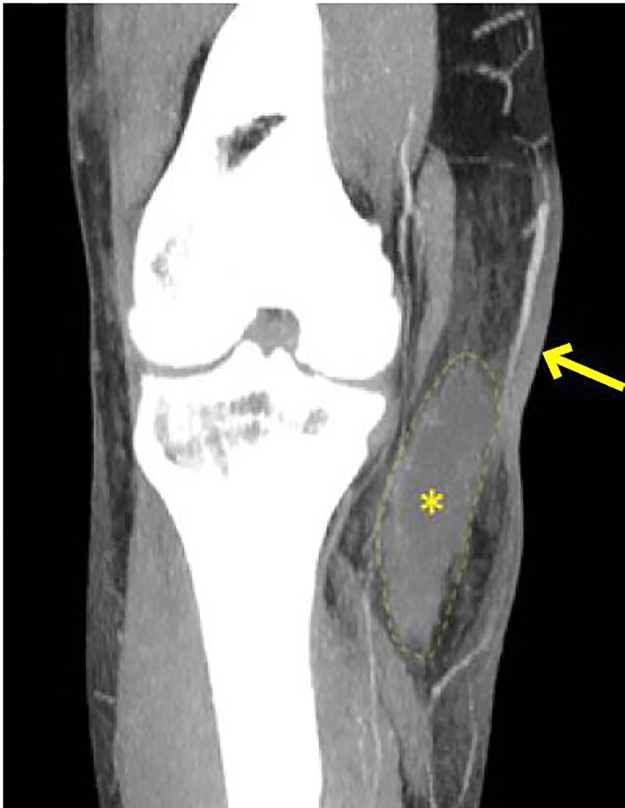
Coronal computed tomography image at level of the knee joint demonstrates an abscess (dashed outline with asterisk) in the medial subcutaneous tissues superficial to the muscle planes. The overlying skin thickening (arrow) reflects cellulitis.
